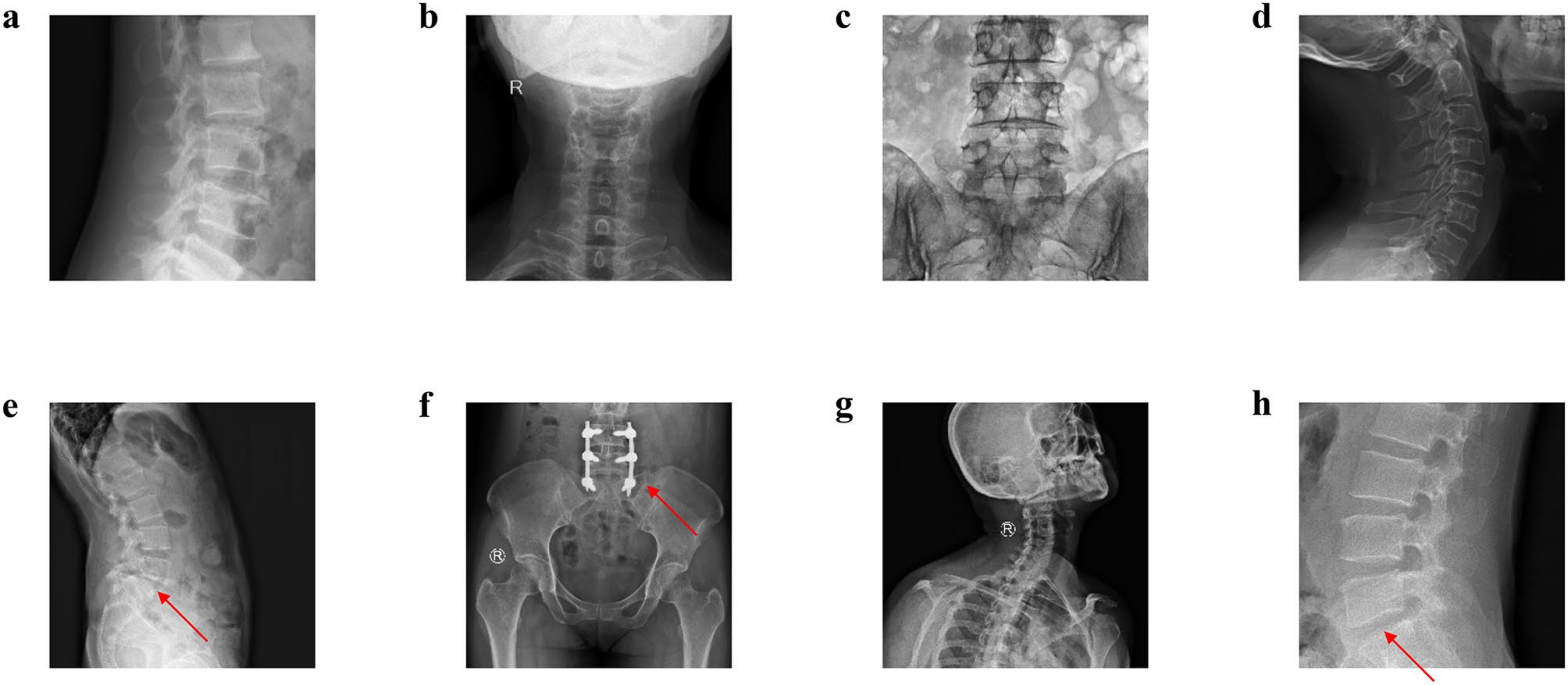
Real spine radiographs used to train the abnormality classifier on real data and train the generative models (GAN and SpineGAN), (a–d, g) No finding, (e) spondylolisthesis, (f) surgical implant), (h) disc space narrowing.
